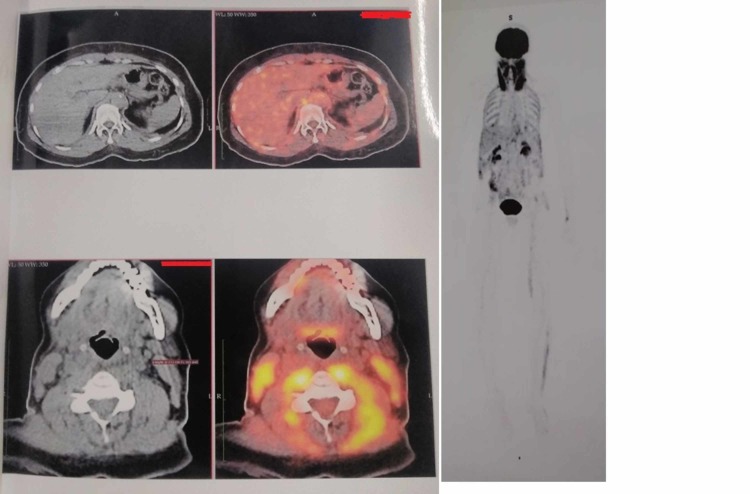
PET CT findings. PET: positron emission tomography
